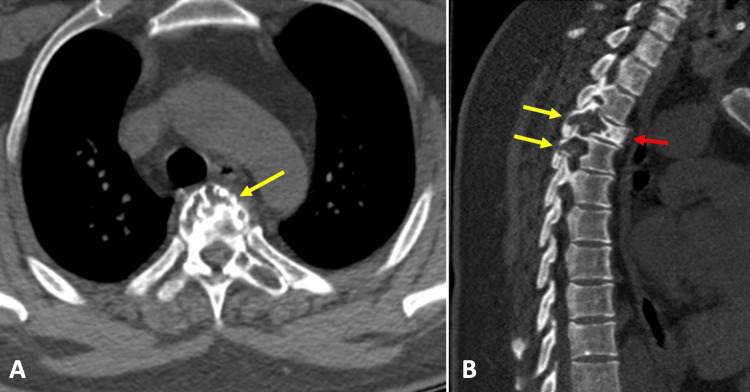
Pre-surgical computed tomography (A) Axial section shows a lytic lesion affecting the vertebral body of T3 (yellow arrow); (B) Sagittal section shows involvement of articular facets and laminae of T3 and T4 (yellow arrows) and decrease in T3 signal (red arrow).
